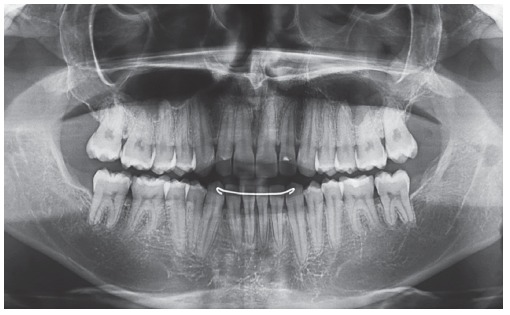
Final radiographic aspect.
